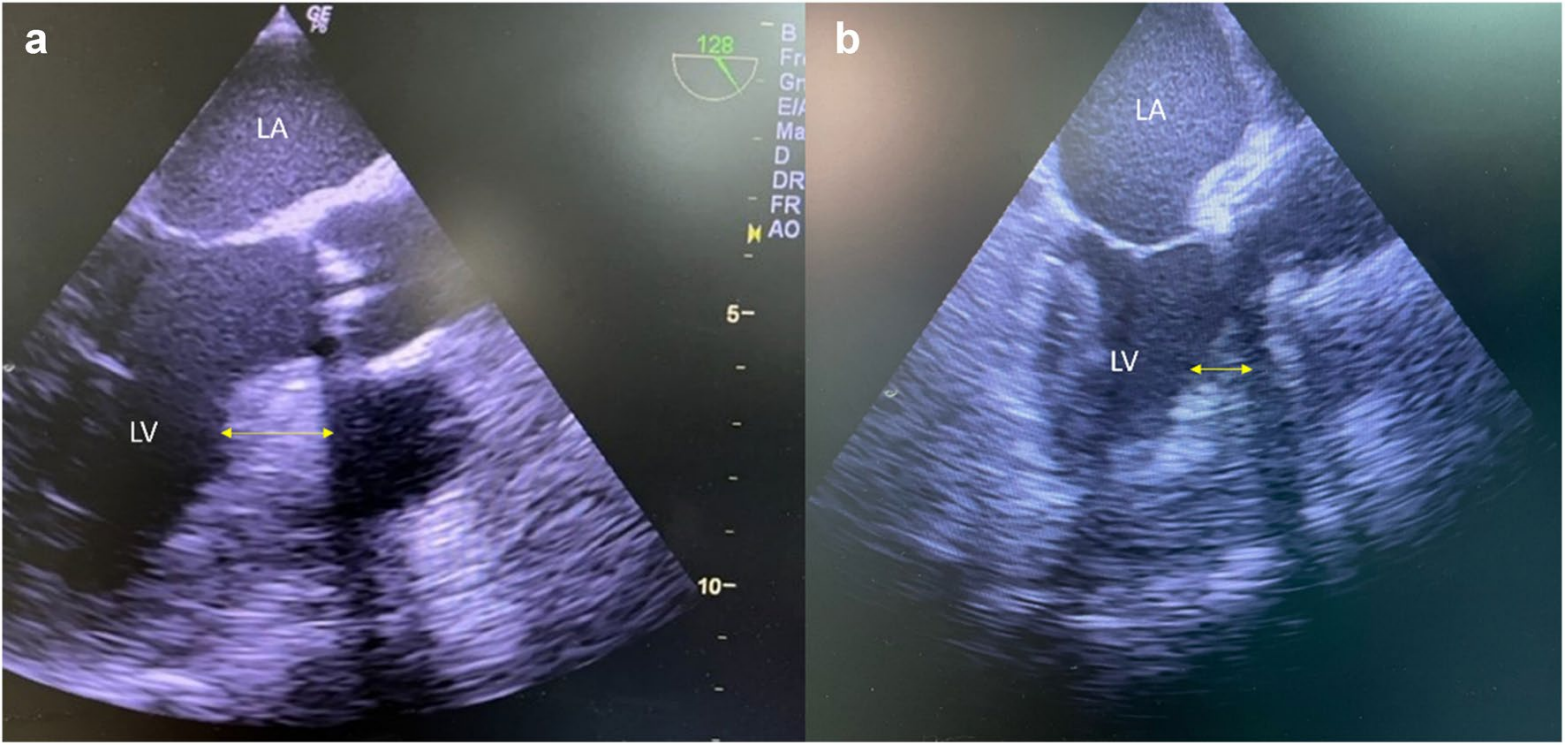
Intra-operative transoesophageal echocardiography a before and b after concomitant septal myectomy. The yellow arrows highlight the diameter of the interventricular septum, which measures 17 mm in a and 7 mm in b
